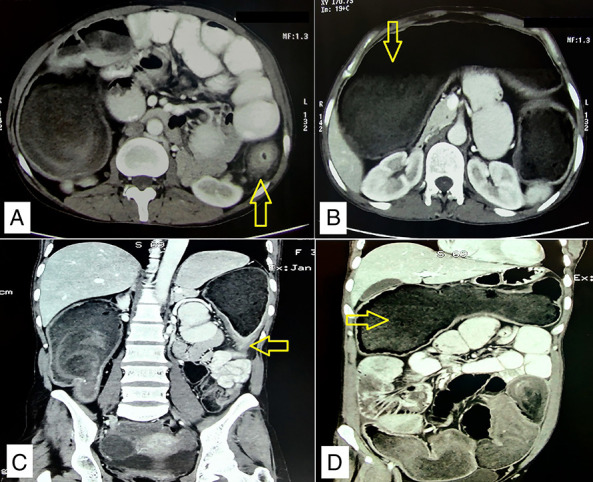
Contrast-enhanced computed tomography of the abdomen and pelvis. (A and B) Axial images showing descending colon stricture (arrows) with dilated proximal bowel. (C and D) Coronal images showing descending colon stricture with dilated transverse colon.
